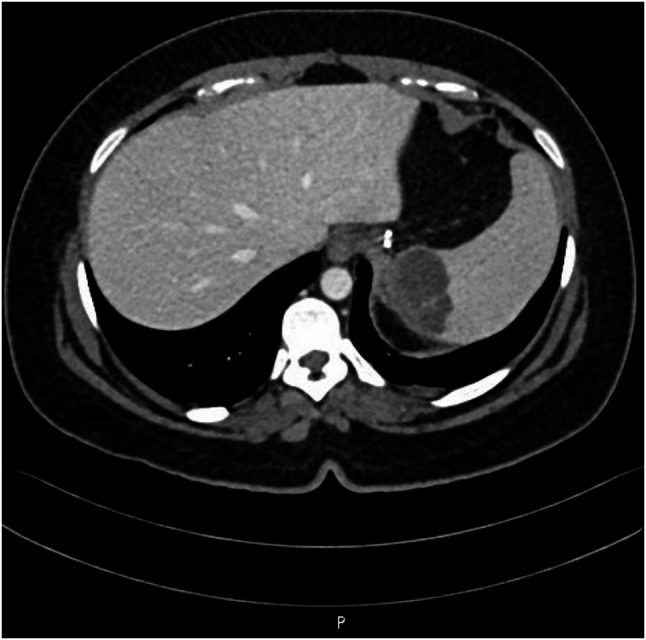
Abdominal CT scan showing a 5 × 2.5 cm hypodense lesion with septation and subtle peripheral irregular enhancement in the superomedial part of the spleen
